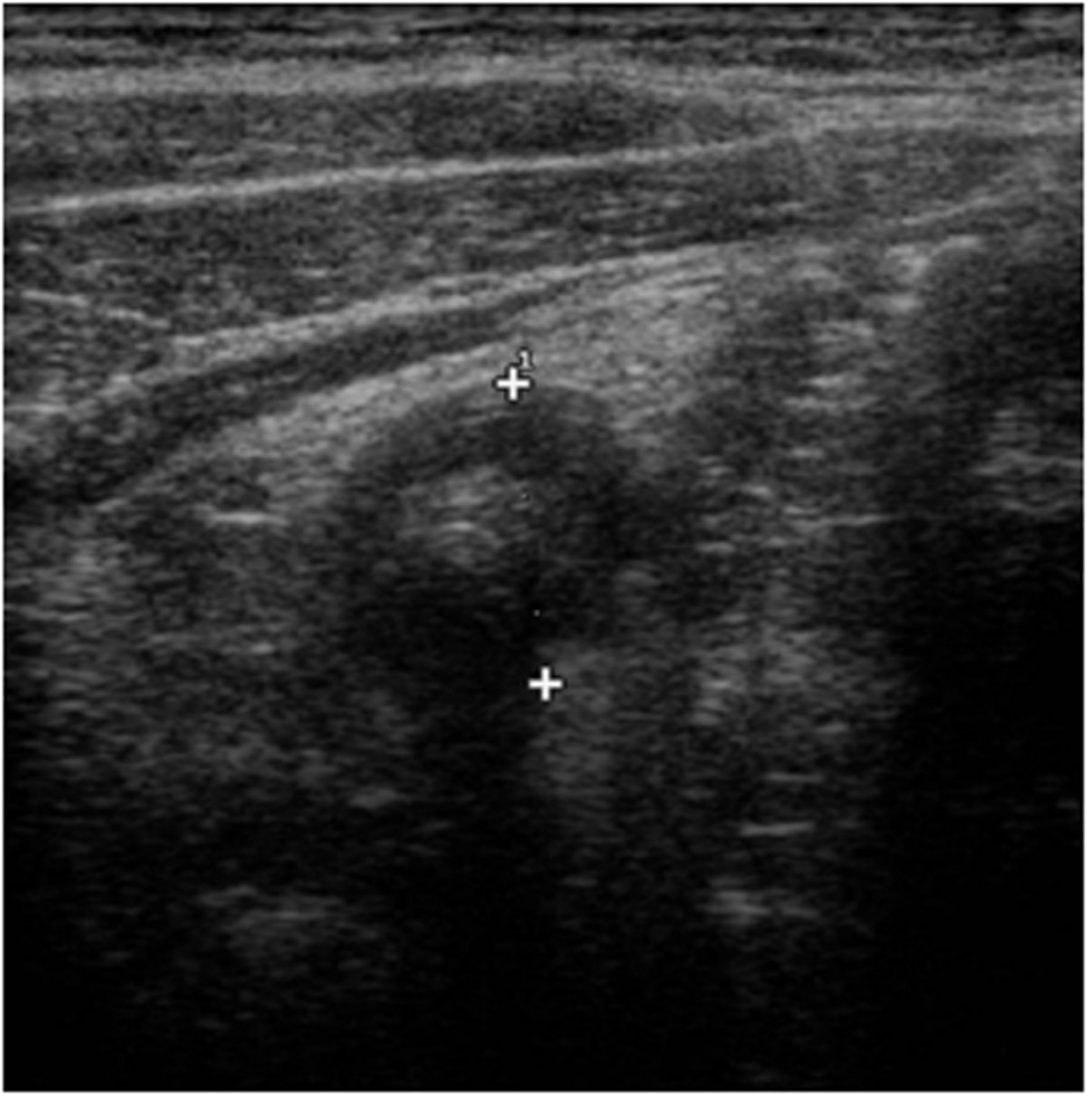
Ultrasound features of appendicitis. On the axial image, we valuated a “target sign” characterized by a fluid-filled center and surrounded by a echogenic mucosa and sub-mucosa and hypo-echoic muscularis.
